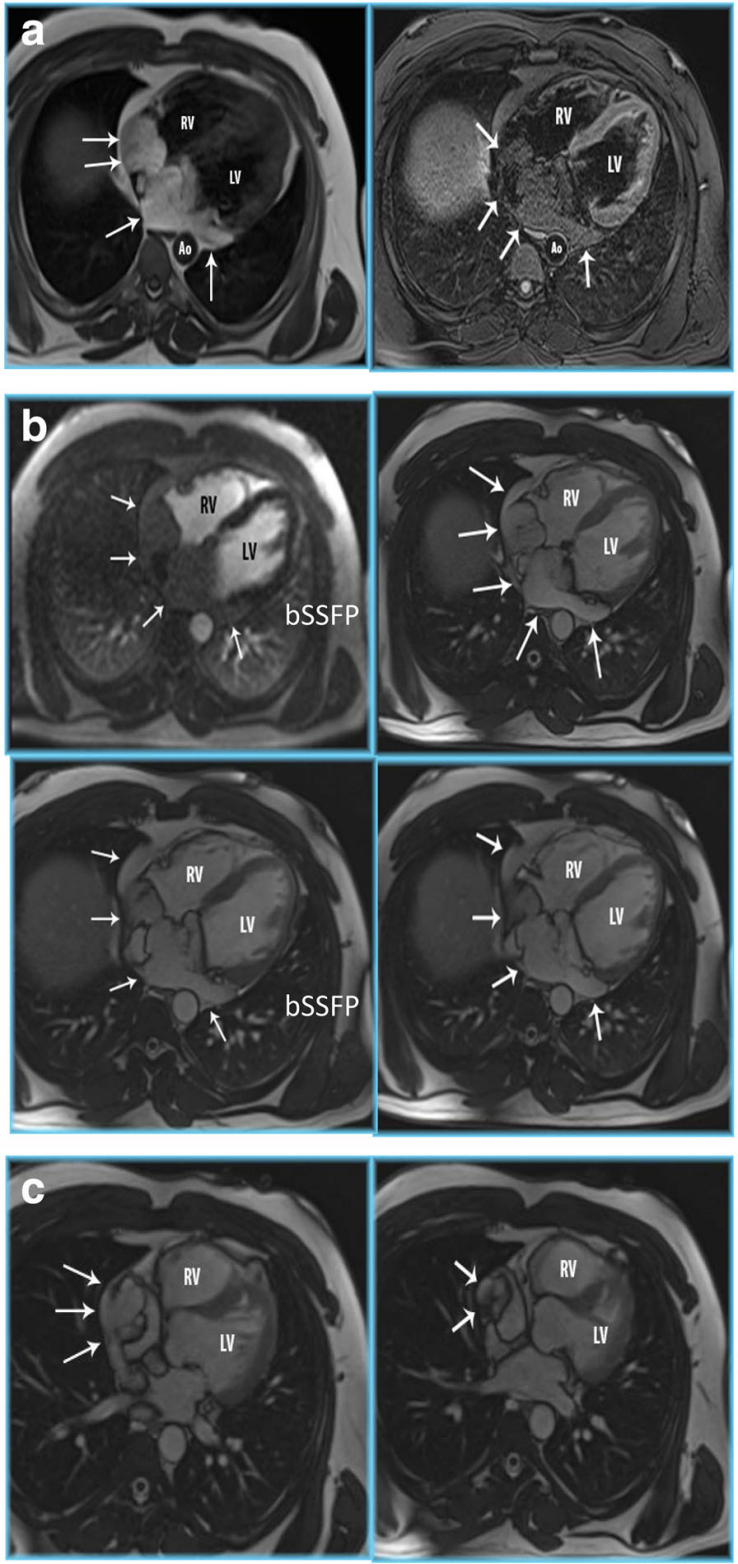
Cardiac MRI of the cardiac lipoma. a Cardiac MR double (DIR) and triple inversion recovery (TIR) sequences clearly demonstrates a large fat containing mass in right atrium, interatrial septum and coronary sinus (*white arrows*). (RV = right ventricle; LV = left ventricle; Ao = descending aorta). b Axial T1 postcontrast (T1 w) and balanced steady state free precession (bSSFP) cardiac MR demonstrates a large fat containing mass in the right atrium, interatrial septum and coronary sinus (*white arrows*). No enhancing components suggests this is a simple lipoma (RV = right ventricle; LV = left ventricle). c Balanced steady state free precession CMR images in HLA shows the lipoma extending from the right atrium into the IVC (*white arrows*). (RV = right ventricle; LV = left ventricle)
